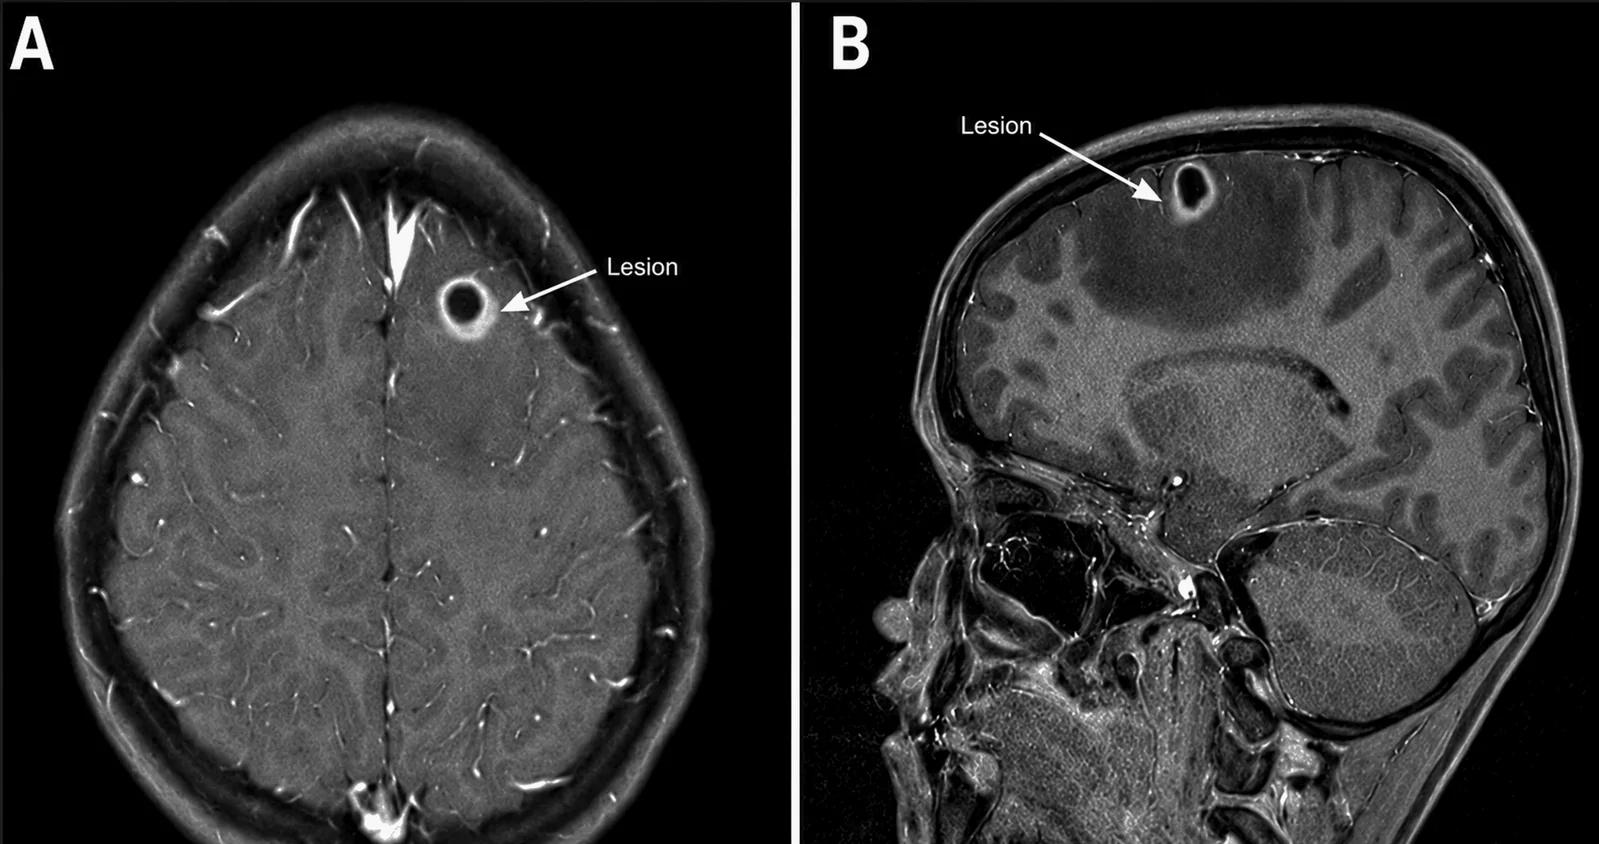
Representative Post-contrast Magnetic Resonance Imaging Appearance of an Intracranial Ring-Enhancing Lesion (A) Axial post-contrast T1-weighted image demonstrates a small ring-enhancing lesion in the cerebral parenchyma, highlighted by the arrow. (B) Sagittal post-contrast T1-weighted image shows the corresponding high frontal convexity ring-enhancing lesion, highlighted by the arrow. These are original anonymized images from the present study. No image has been reused or adapted from a published source. All patient-identifying information has been removed.
